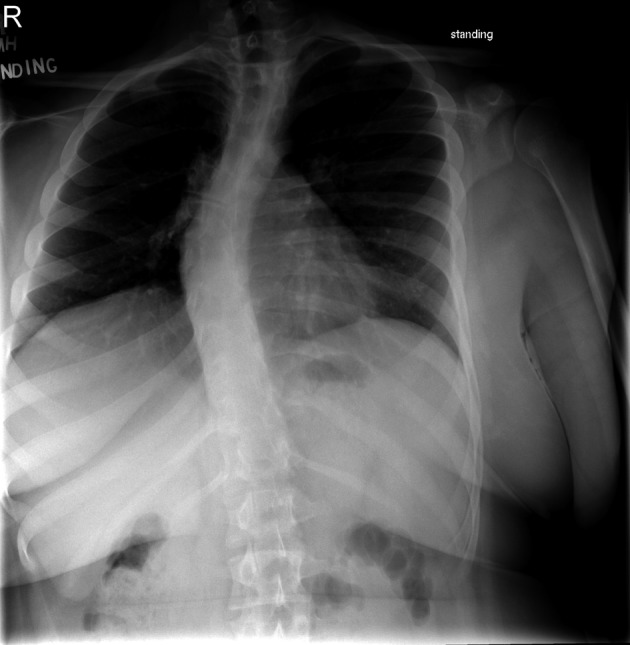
AP and lateral thoracolumbar spine X-Rays demonstrating S-shaped scoliosis of the cervical and thoracic spine with left lower cervical and upper thoracic curves and right mid and lower thoracic curves. Increased thoracic kyphosis and lumbar lordosis is present. There is mild anterior wedging of a mid thoracic vertebra.
